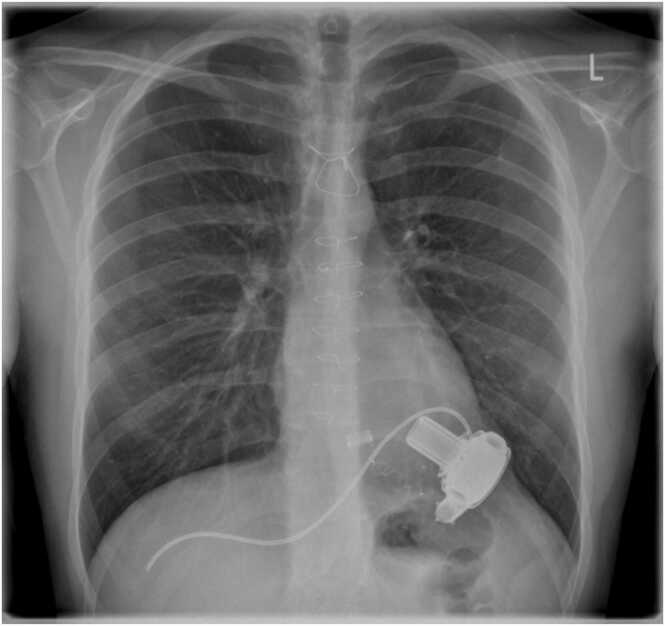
The most recent chest X-ray (CXR) showing a normal cardiothoracic ratio with the presence of the decommissioned HeartWare HVAD.
